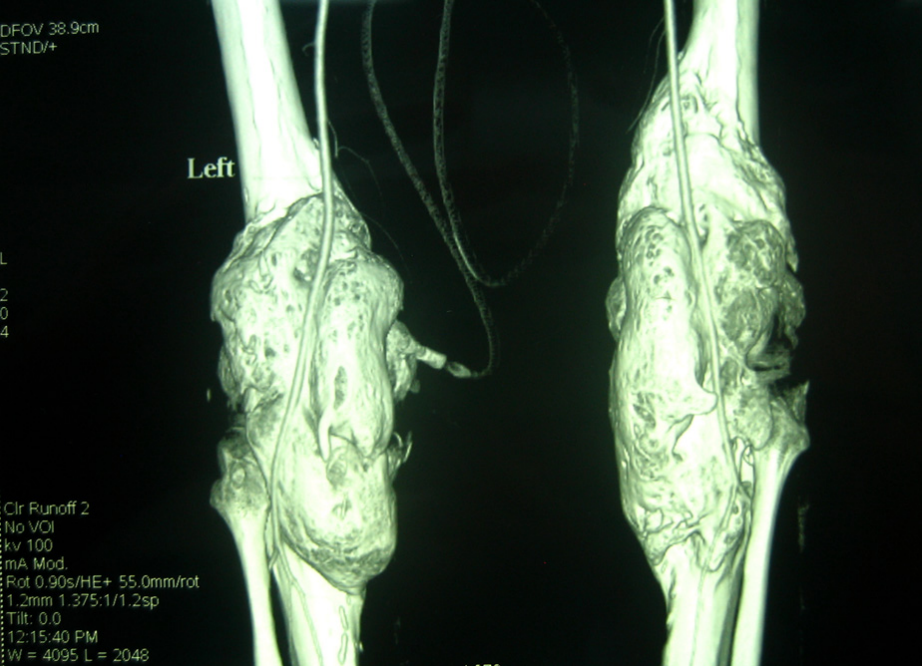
CT Angiography of the knees; both popliteal arteries encased in grooves of the heterotopic bone.
